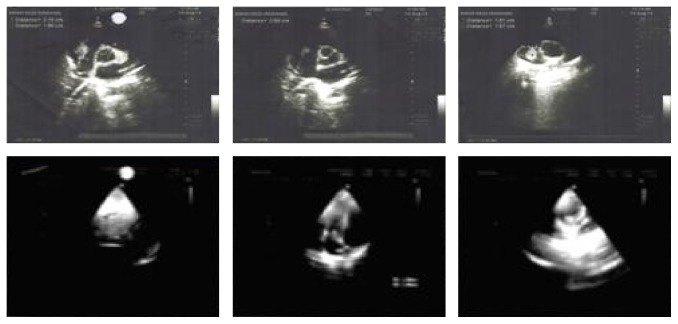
Transesophageal echocardiography (TEE) of a right atrial mass in the patient.
